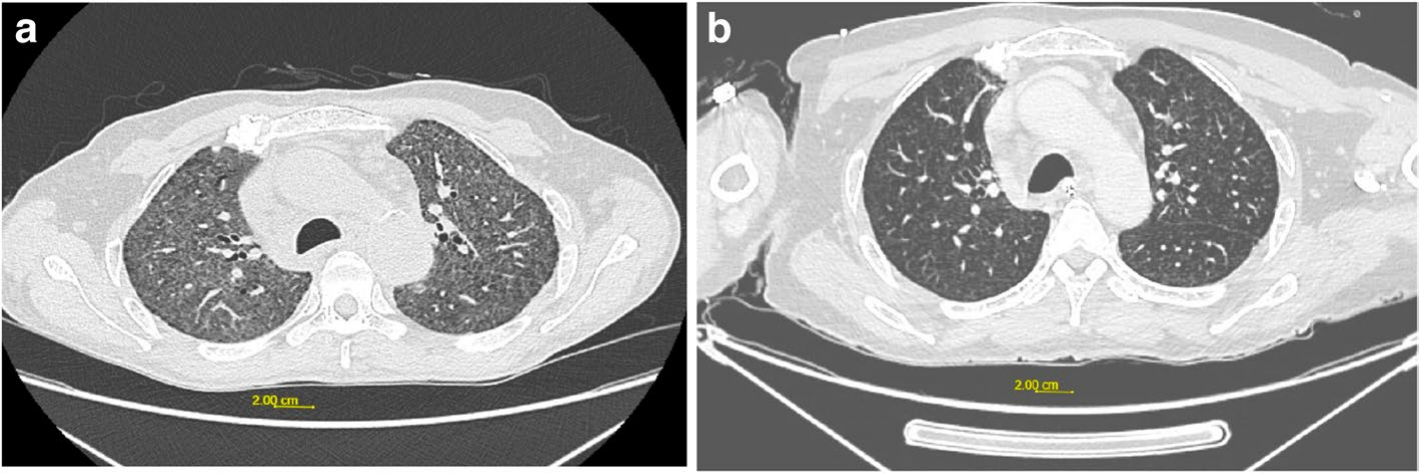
a: Initial chest CT scan of case 2 showing diffuse ground glass lesions. b: 6-month follow-up CT scan showing improvement of ground glass lesions
